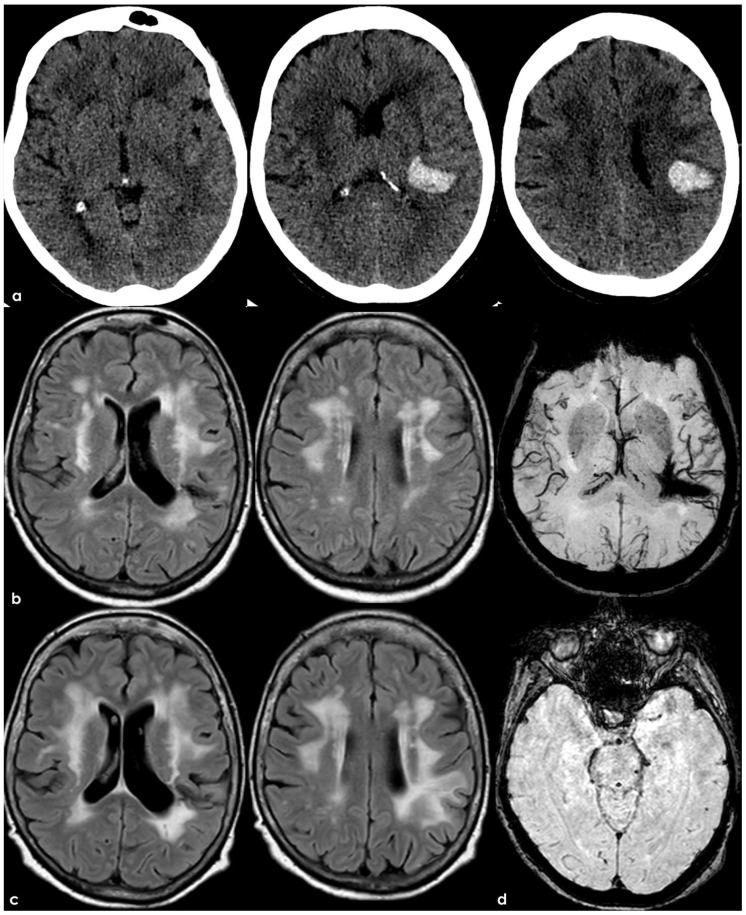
Non-contrast CT (NCCT) and MRI findings in a patient with CAA-related intracerebral hemorrhage (ICH), and CAA-related inflammation (CAA-ri). Panel (a): Axial NCCT images (displayed from inferior to superior, left to right) demonstrate confluent symmetrical white matter hypodensities consistent with small vessel disease and a hyperdense acute intracerebral hemorrhage located at the left temporo-parieto-frontal junction. Panel (b): Axial FLAIR MRI obtained three months later confirms confluent, symmetrical supratentorial white matter hyperintensities (WMHs). Panel (c): Axial FLAIR MRI performed two years later reveals a left posterior frontal white matter hyperintensity with mild mass effect, consistent with spontaneous ARIA-E. The MRI was acquired following new-onset refractory status epilepticus (NORSE), supporting the clinical suspicion of CAA-ri. Panel (d): Axial susceptibility-weighted imaging (SWI), reconstructed using a minimum intensity projection (minIP) protocol, shows the residual hypointense cavity from the prior ICH and a single left temporal cerebral microbleed.
